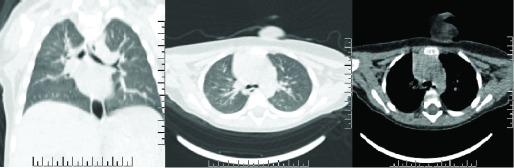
Repeat CT scan of the chest showing infection of the right upper lobe and narrow right upper lobe bronchus.
